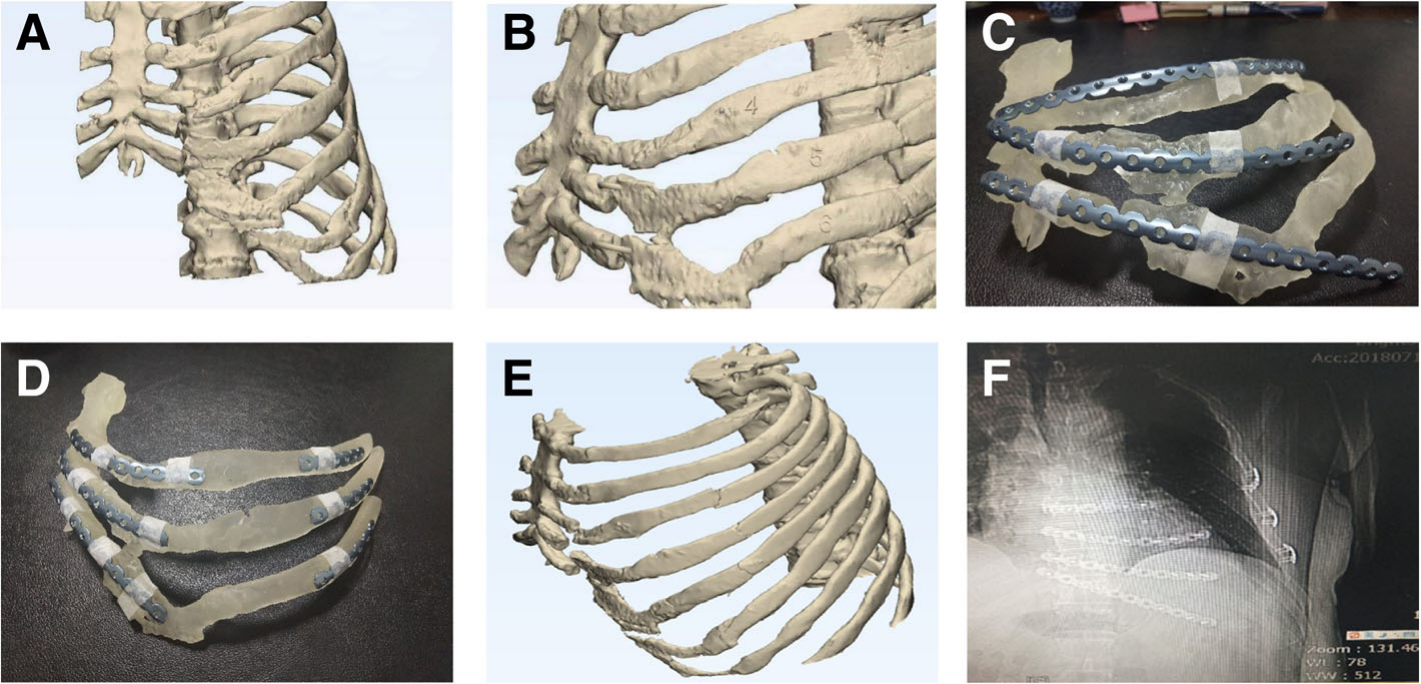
No.2 patient (a) According to the 3D model made by CT, 4 and 5 costal cartilage fractures and 6 costal anterior costal arch fracture can be seen. b The 3D model of the fracture end of the rib was adjusted by using 3D software model. c The 3D printed 4–6 rib model shows a large gap between the locking plate and the shape of the rib. d After giving the shape, the locking plate and the rib paste are in good condition. e, f Postoperative review, the shape of the internal fixator was intact, and the shape of the contralateral rib recovered well compared with the preoperative image
